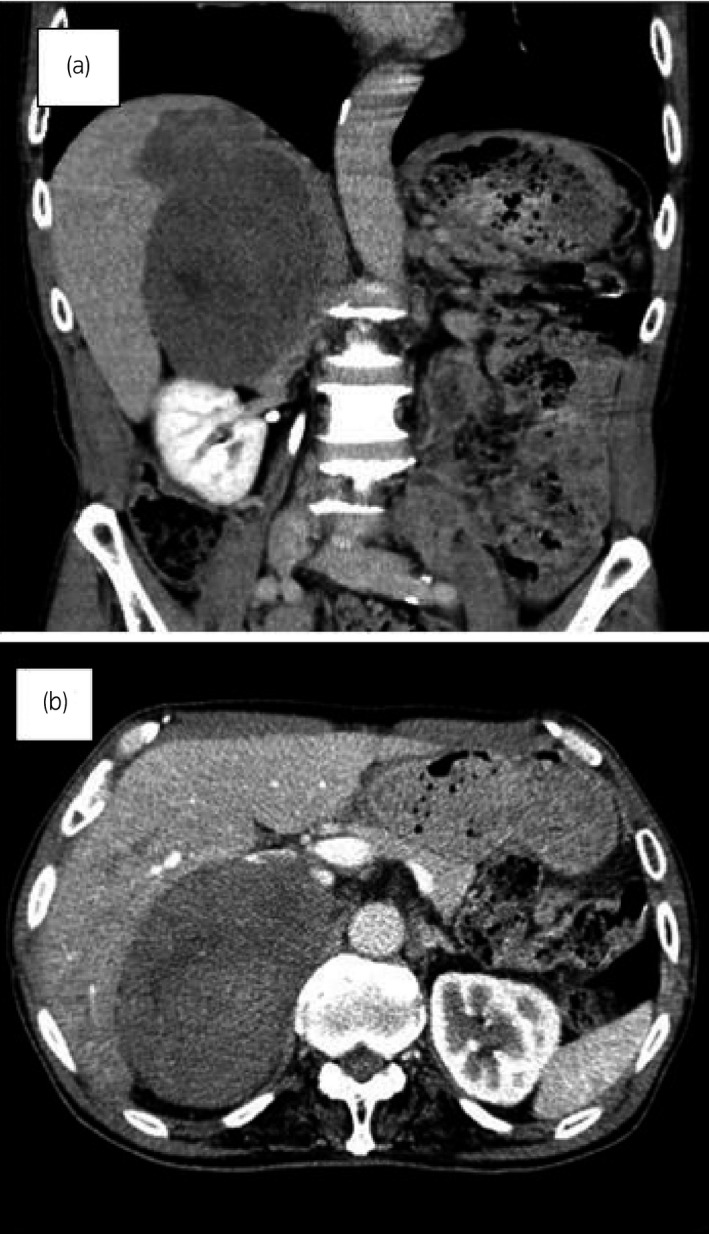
CT of the abdomen (a: coronal image, b: axial image). A huge adrenal mass with a maximum diameter of 15 cm which extended to the right liver lobe.
